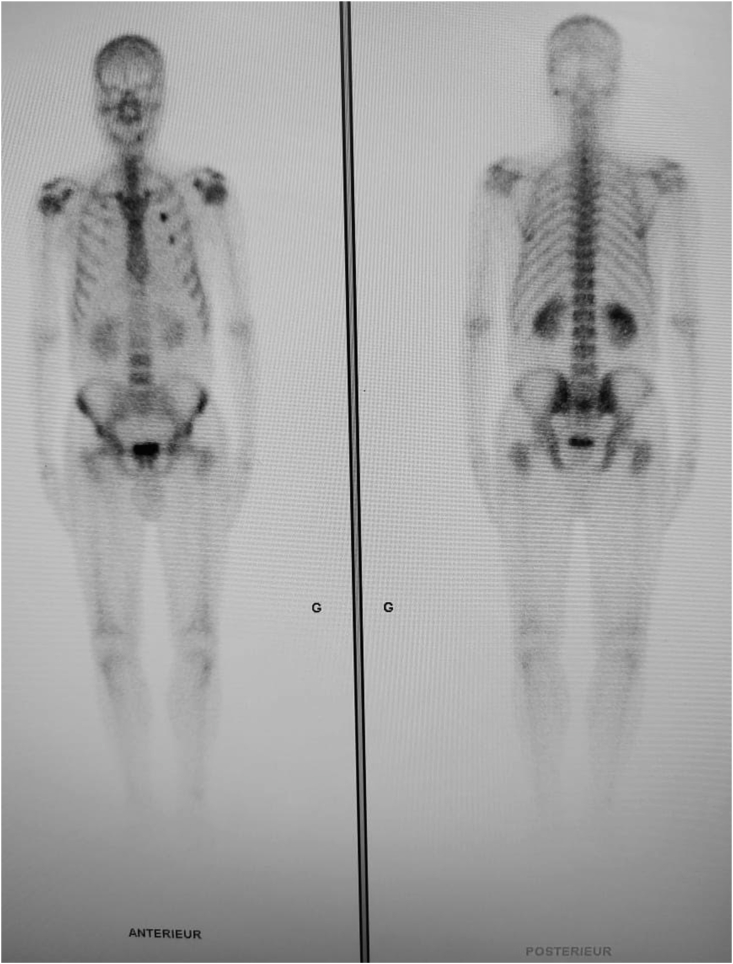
Bone scintigraphy showing the absence of cardiac uptake and suggestive of light-chain cardiac amyloidosis.
